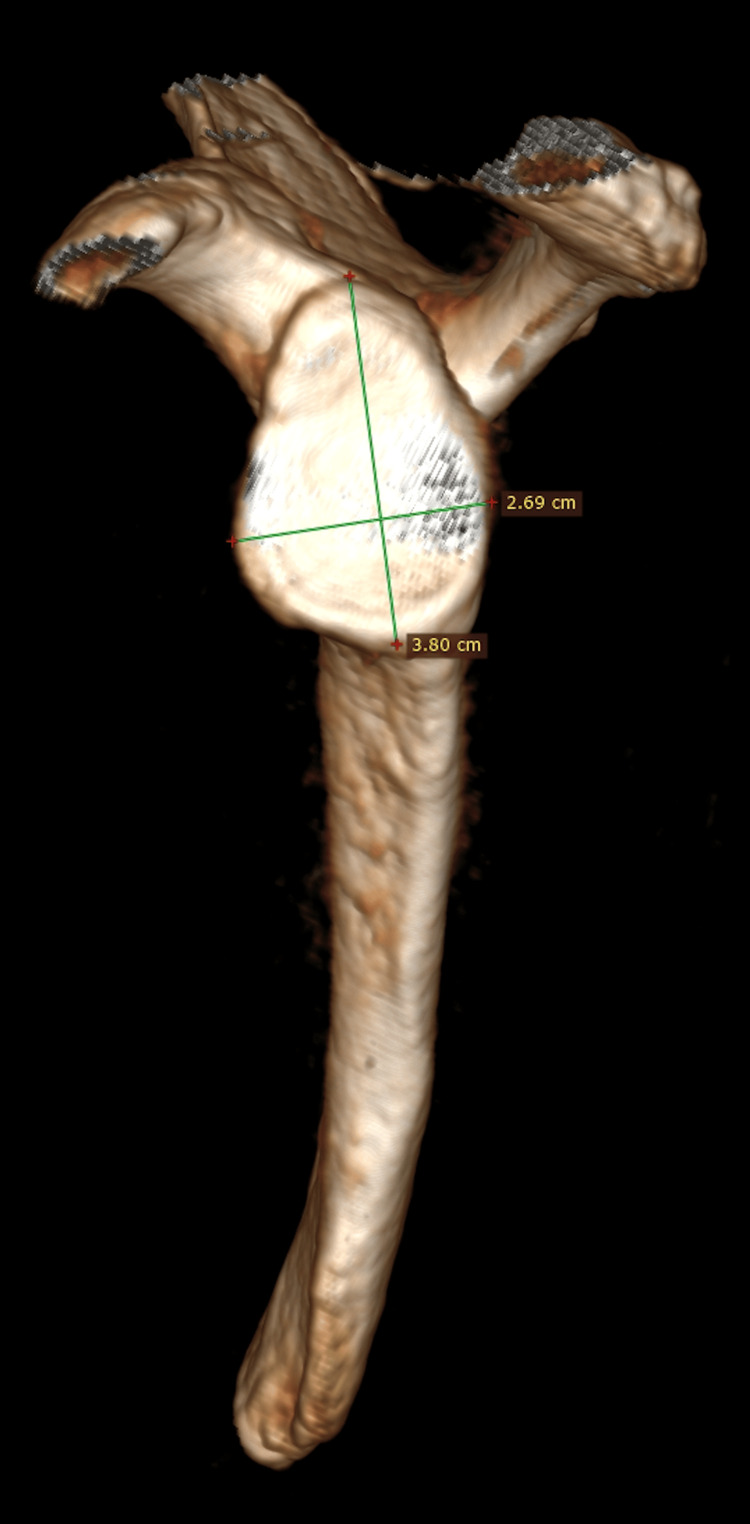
A 3D CT reconstructed model of the glenoid. A 3D CT reconstructed model of a right glenoid. Measurement of the maximum superoinferior length and the maximum anteroposterior width is possible.
